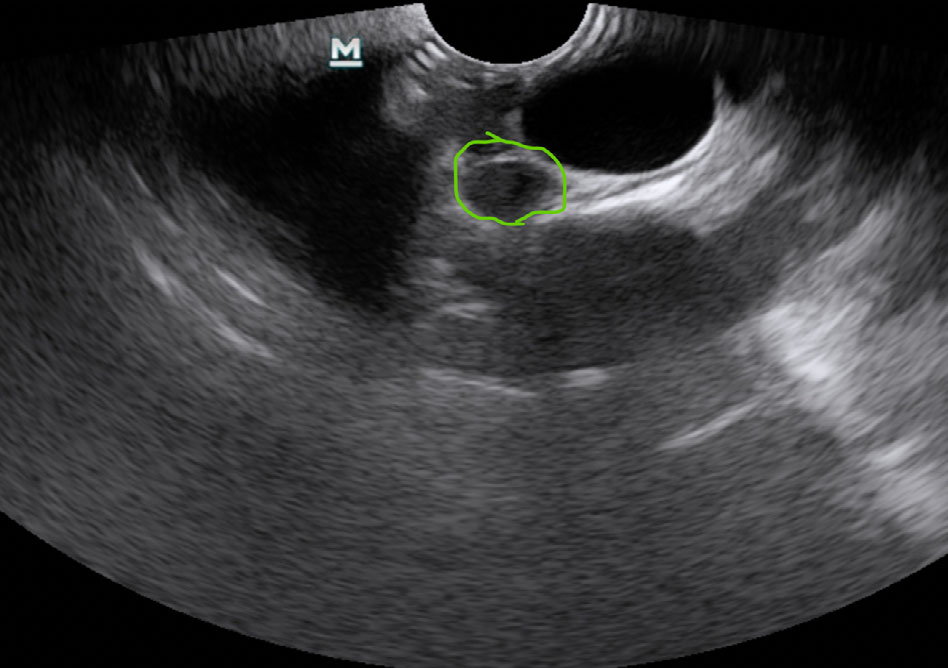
Pathological nodal sonographic characteristics round shape, the absence or presence of central hilar structure, increased color Doppler flow.
